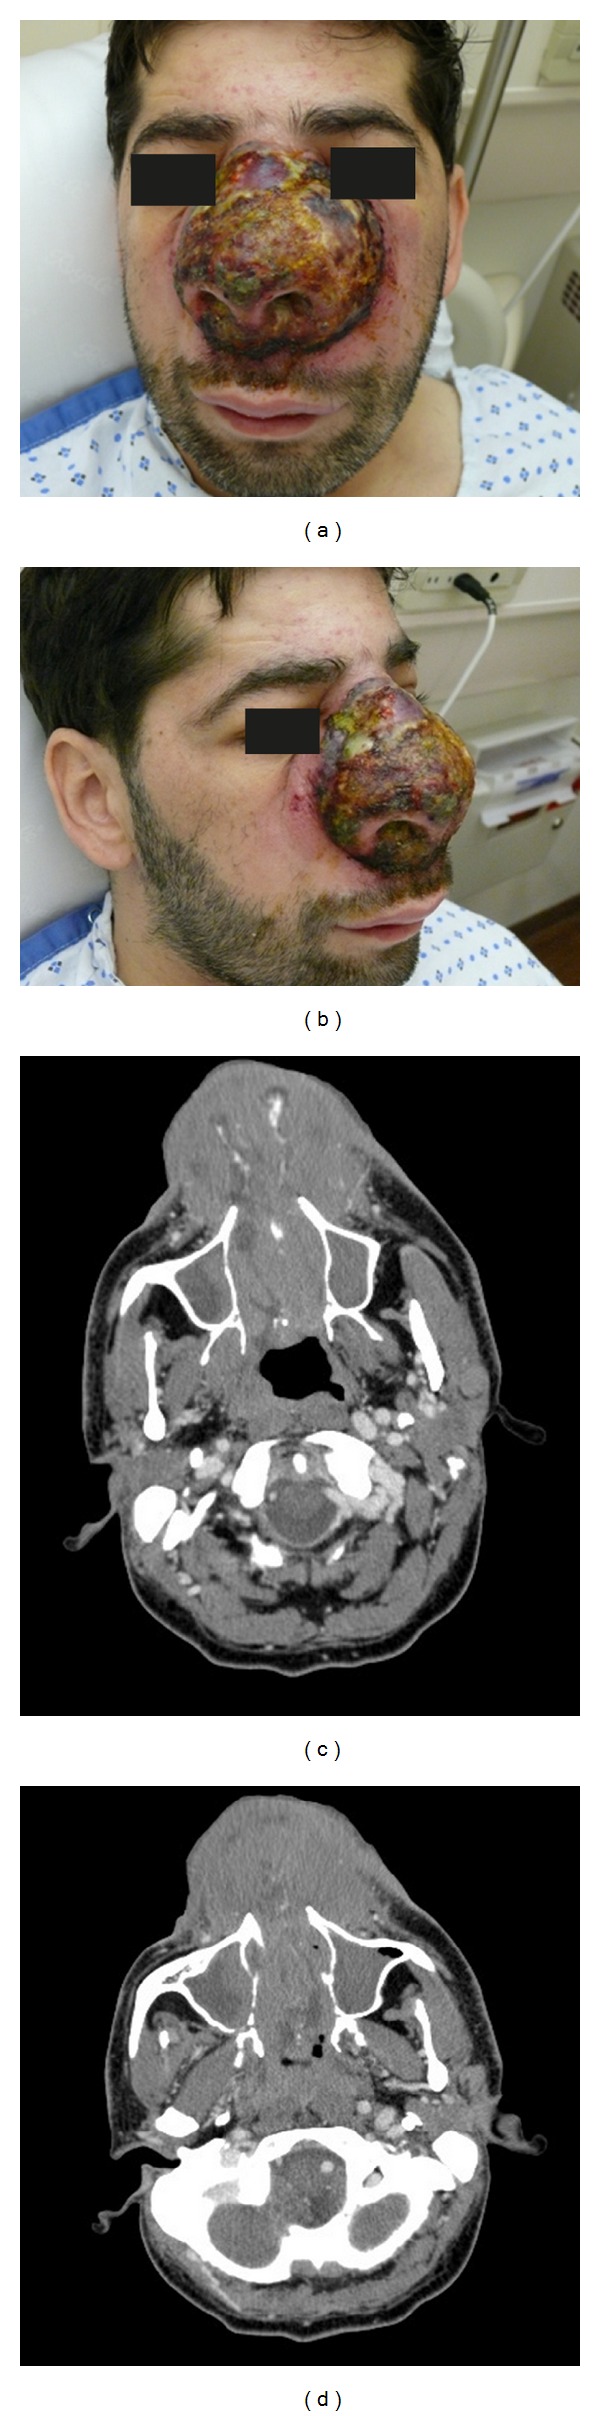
Pictures (a) and (b) showing anterior and lateral views of nasal granuloma from metastatic testicular ENKTCL-N lymphoma. Pictures (c) and (d) showing a CT of the sinuses with and without contrast, respectively, showing the nasal mass. Note: the patient had consented for the use of those pictures in research purposes.
